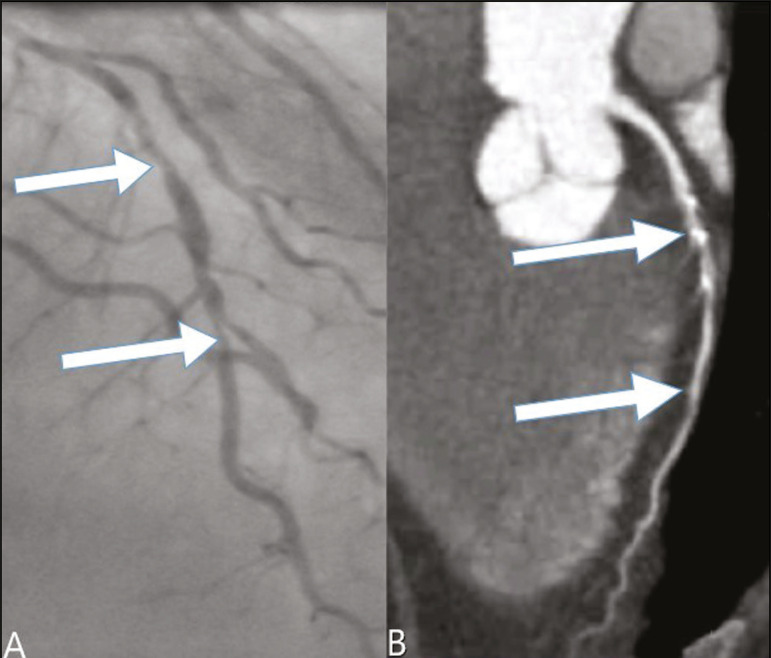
Mixed plaque, resulting in 70% stenosis in the proximal segment of the ADA, and noncalcified plaque, resulting in 90% stenosis in the distal segment of the ADA (CAD-RADS 4A). A: Analysis by catheterization. B: Analysis by CTA.
